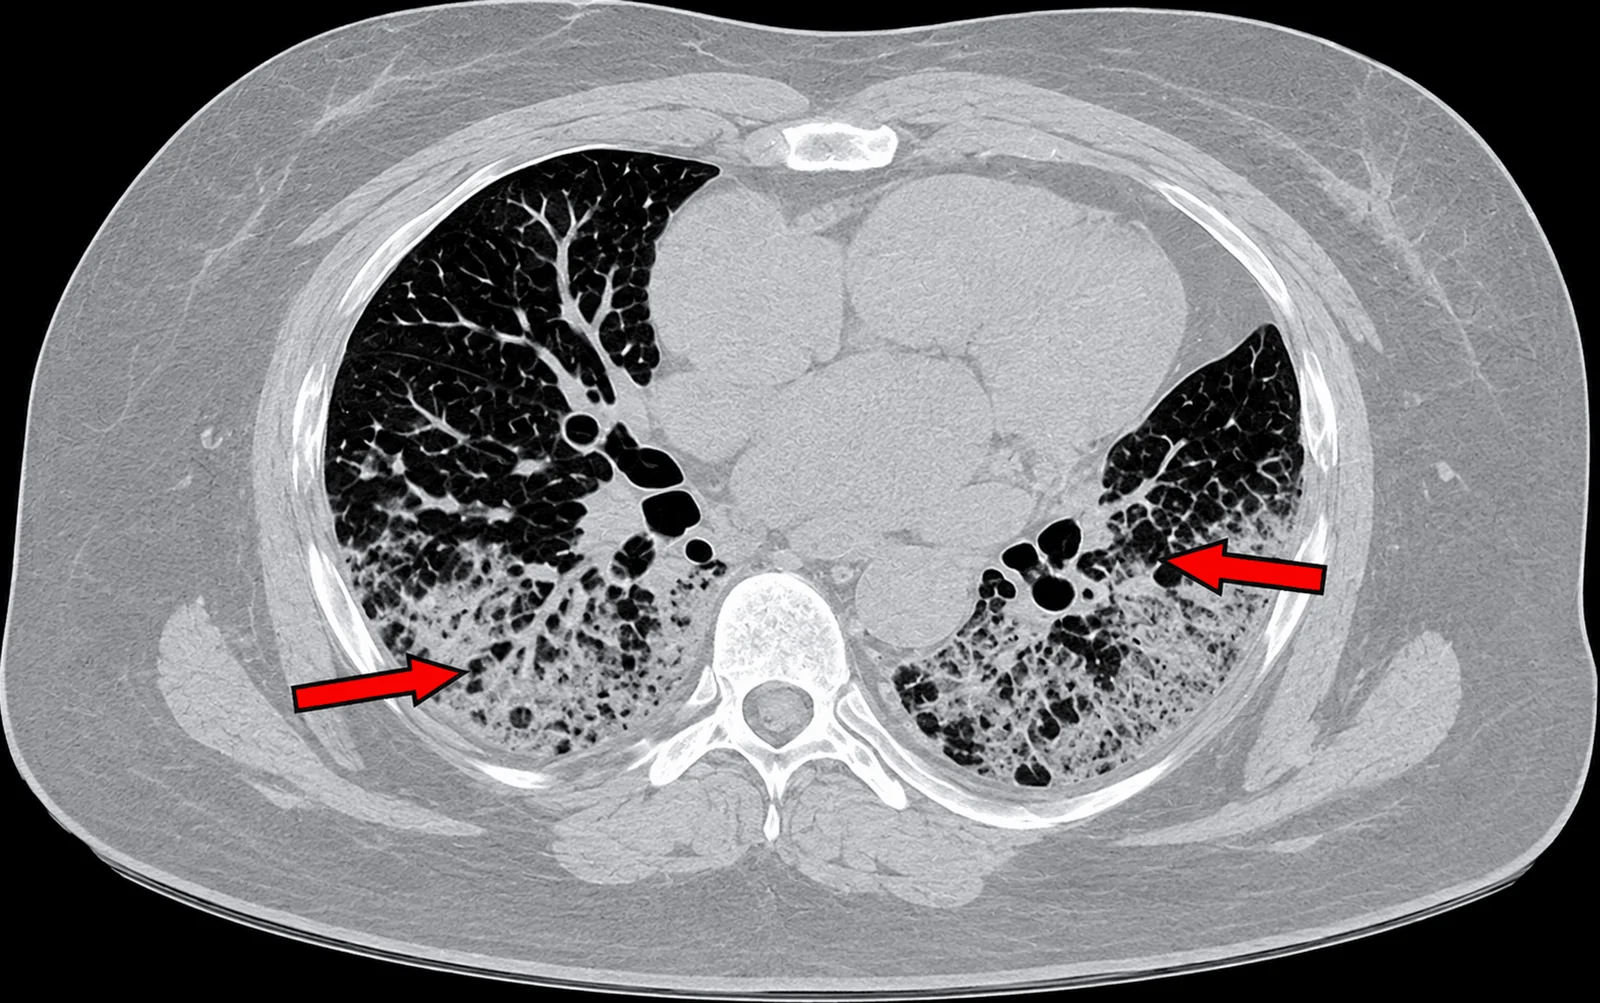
High-resolution computed tomography (HRCT) of the chest showing opacities with underlying bronchiectasis and thickened interlobular septum (arrows) involving both lungs
